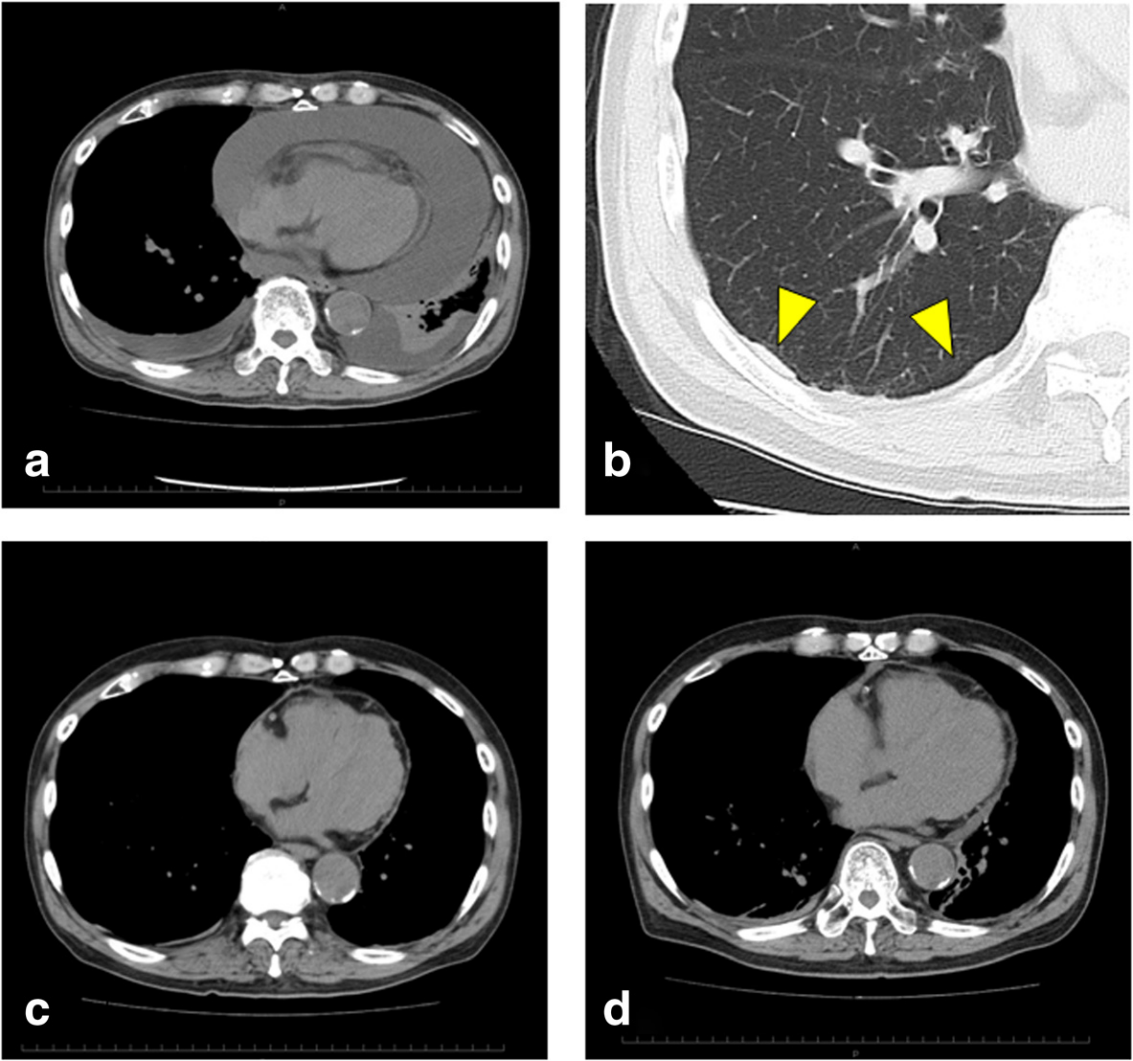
Findings from chest computed tomography. A chest computed tomography (CT) revealed massive pericardial effusion and bilateral pleural effusions on admission (a). A chest CT after 8 months clearly showed pleural plaques (b
